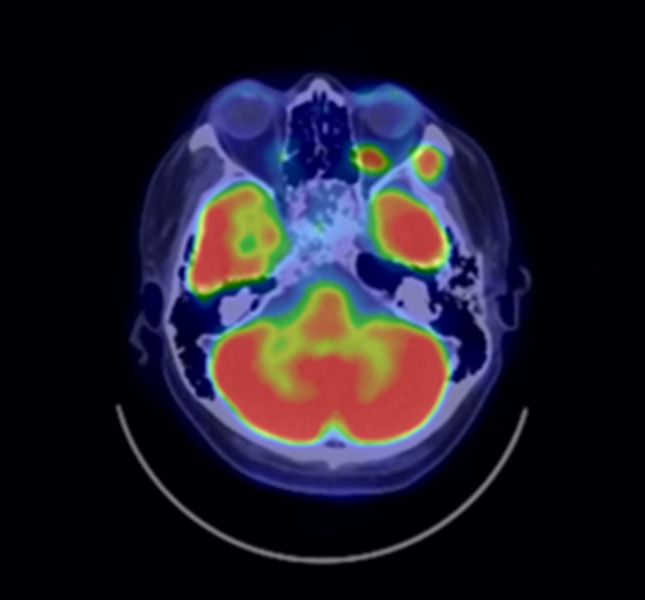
PET-CT after proton beam therapy (PBT). PBT resulted in the disappearance of fluorodeoxyglucose in the sphenoid sinus
